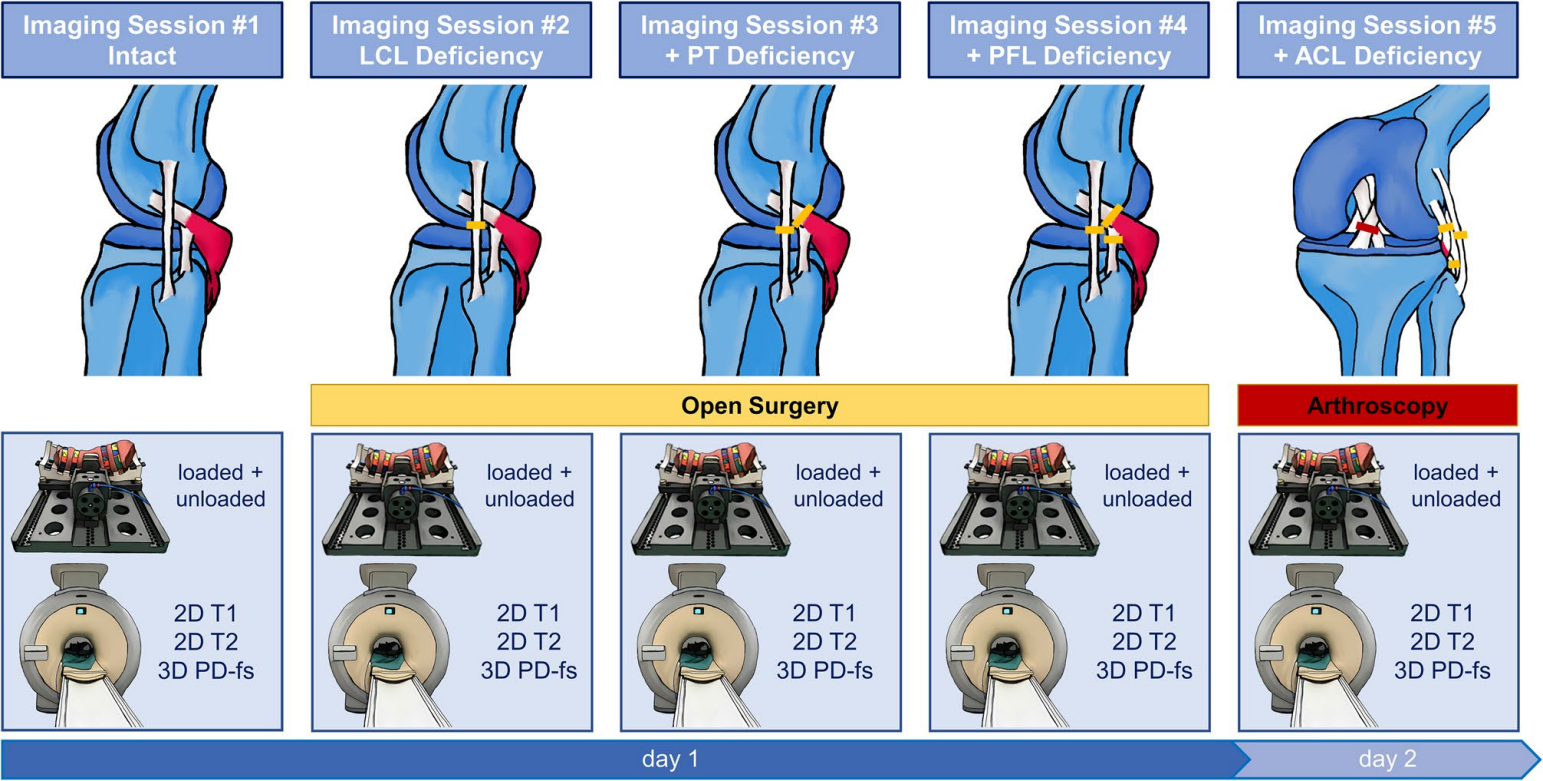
Timeline of imaging sessions as a function of graded injury. The lateral collateral ligament (LCL), the popliteal tendon (PT), the popliteofibular ligament (PFL), and the anterior cruciate ligament (ACL) were transected via open surgical (orange) and arthroscopic approaches (red), respectively. T1-weighted, T2-weighted, and proton density-weighted fat-saturated sequences were used to image the joints in each condition and configuration.
